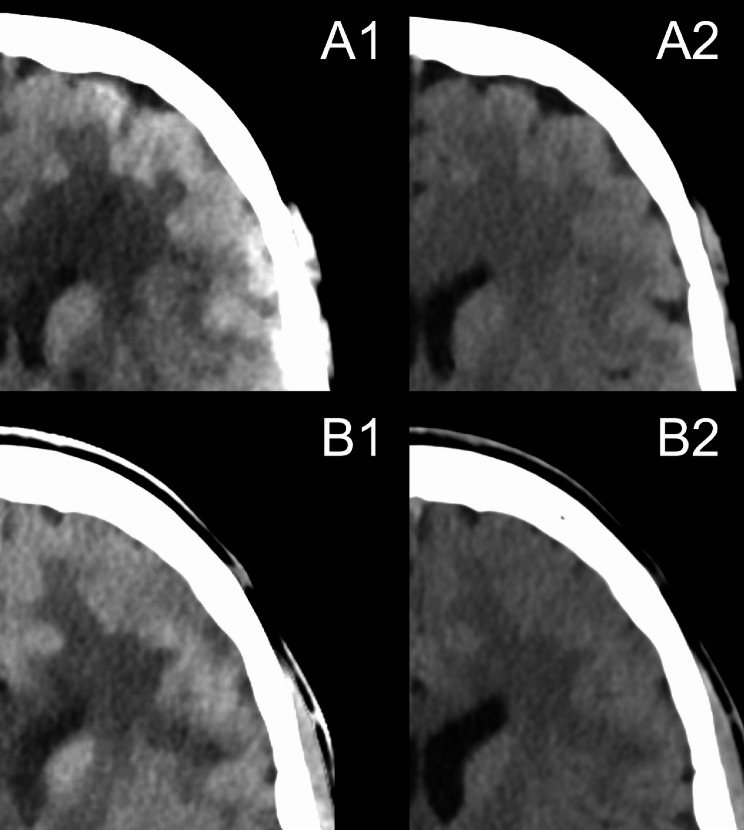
Inhomogeneity of the cortical brain tissue in axial CT images. The VMI 40 keV in VA50A (A1) and VMI 65 keV in VA50A (A2) as well as the VMI 40 keV in VB10 (B1) and VMI 65 keV in VB10 (B2) are shown. The representative section of the cortical brain tissue in VA50 shows the clear differences in attenuation with higher values close to the calvarium, which are particularly apparent in the VMI 40 keV. The brain tissue is displayed inhomogeneously. In VB10, this effect is significantly less pronounced and subjectively barely distinguishable even in VMI 40 keV. All images are displayed with equal window settings (C40/W80). VMI: virtual monoenergetic image; keV: kiloelectronvolt; C: window center; W: window width
